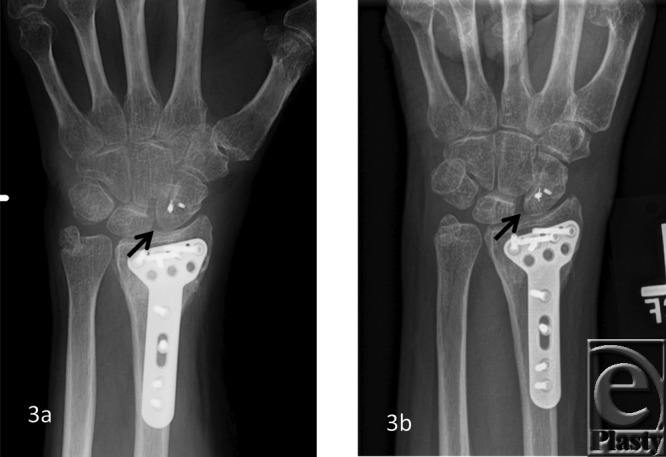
A 50-year-old woman with prior ORIF and SLIL ligament repair. Normal SL space (*arrow*) on posteroanterior X-ray view (*a*). Notice mild gaping of S-L interval (*arrow*) on clenched fist posteroanterior X-ray view (*b*). ORIF indicates open reduction internal fixation; SLIL, scapholunate interosseus ligament.
